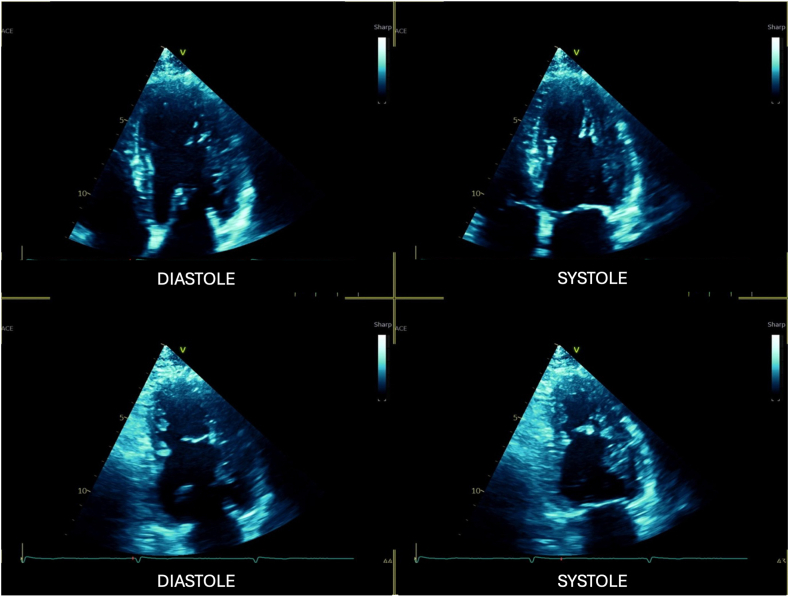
Two-dimensional TTE, apical 4-chamber (*top*) and apical 2-chamber (*bottom*) views in diastole (*left*) and systole (*right*), demonstrates normal LV size with moderately reduced LV global systolic function (LVEF = 37%) without regional wall motion abnormalities; the IVMSA is not visualized in these conventional imaging planes.
